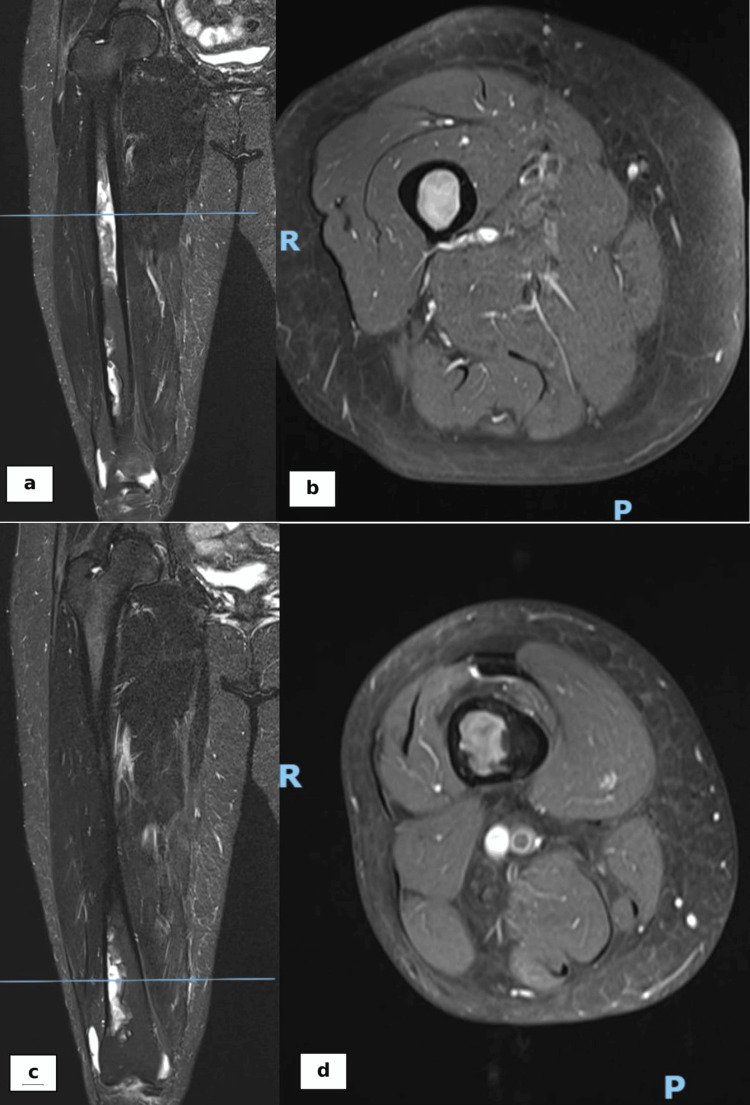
Proximal one-third of the femur in coronal (a) and axial (b) views of the MRI revealed a prominent fatty signal in the femoral shaft without endosteal scalloping, raising suspicion of an intramedullary lipomatous tumor. Similar features were seen on the distal femur in coronal (c) and axial (d) views.
